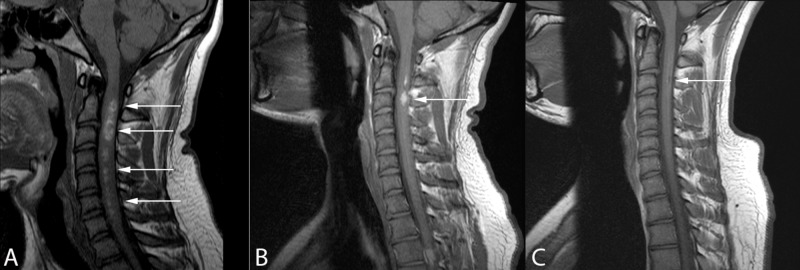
Sagittal T1-weighted MRI at patient presentation (A), three-week follow-up (B), and nine-week follow-up (C) depicting almost complete spontaneous resolution of the cervical intramedullary spinal cord lesion (arrows)
